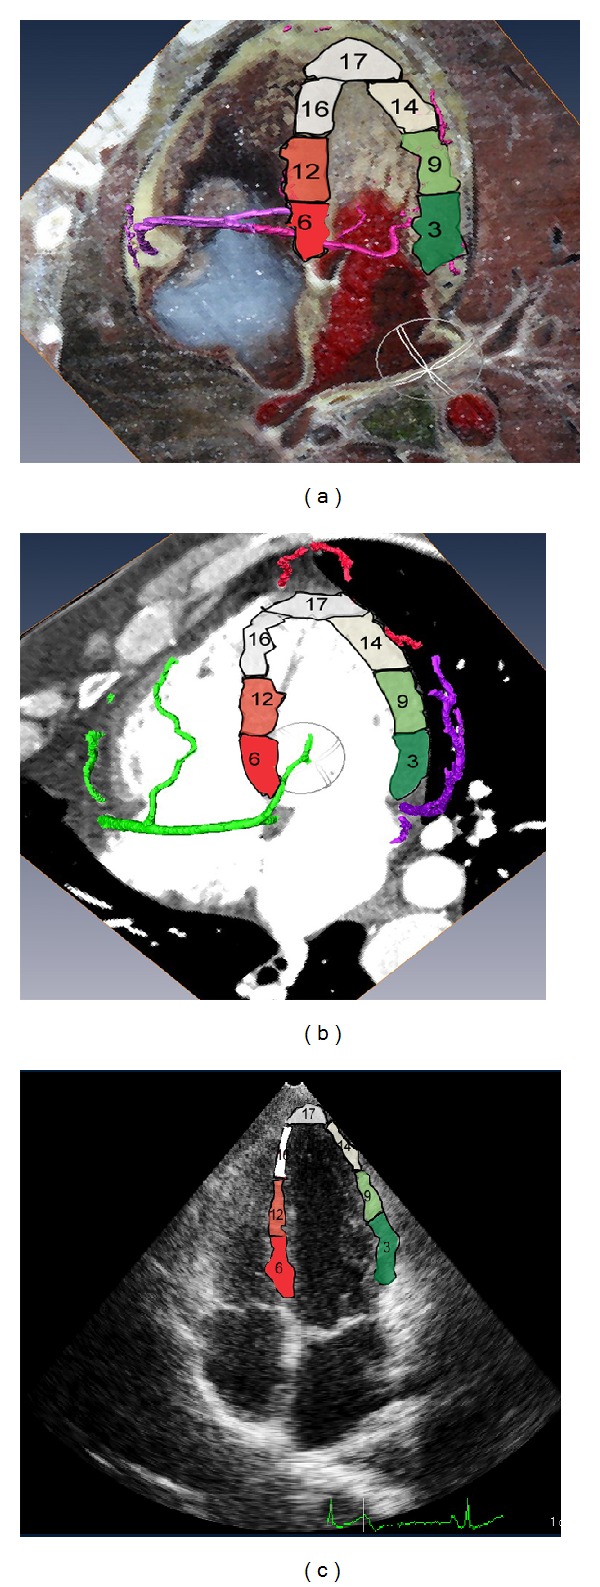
Four-chamber heart sections. (a) Fusion display of the CVH dataset and the coronary artery model. (b) Fusion display of the cardiac CT dataset and the coronary artery model. (c) Two-chamber heart section revealed by echocardiography.
